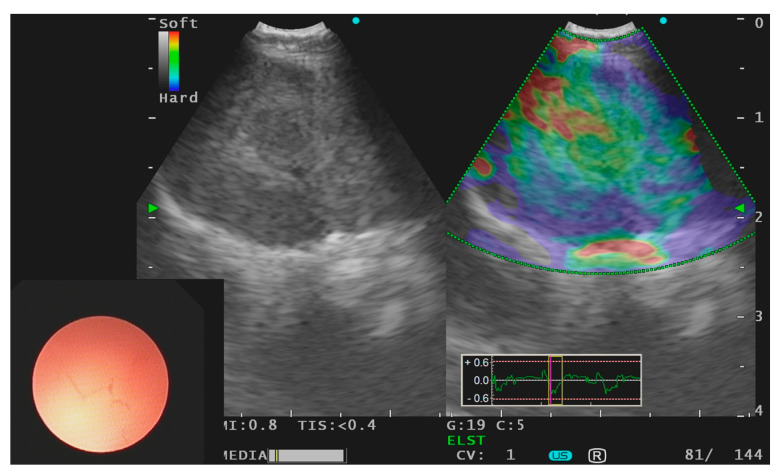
Endobronchial ultrasound using linear probe with use of elastography of a subcarinal lymph node harboring primary lung adenocarcinoma.
